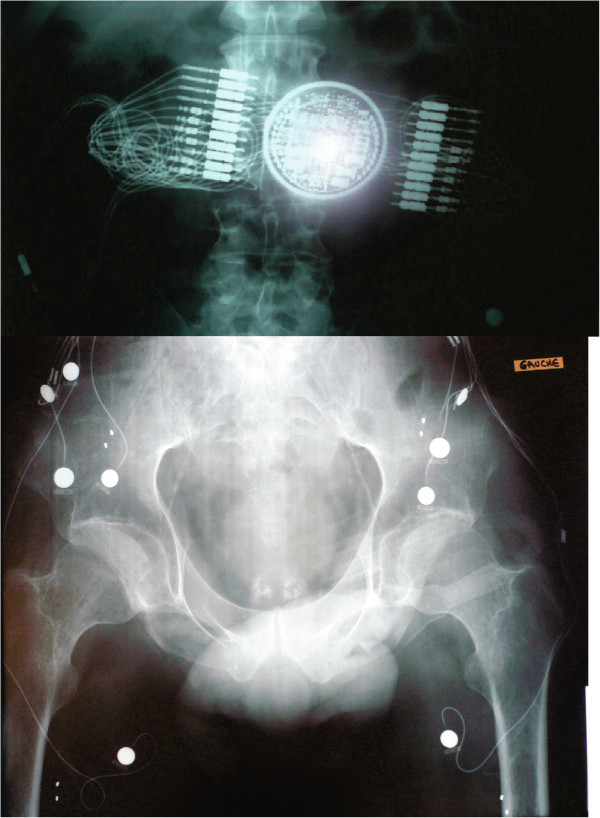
X-ray (face) of the system obtained in 2009 shows no visible damage of the wires, connectors, electrodes or implant electronics. Big white dots are epimysial electrodes, small pair of dots are neural electrodes.
